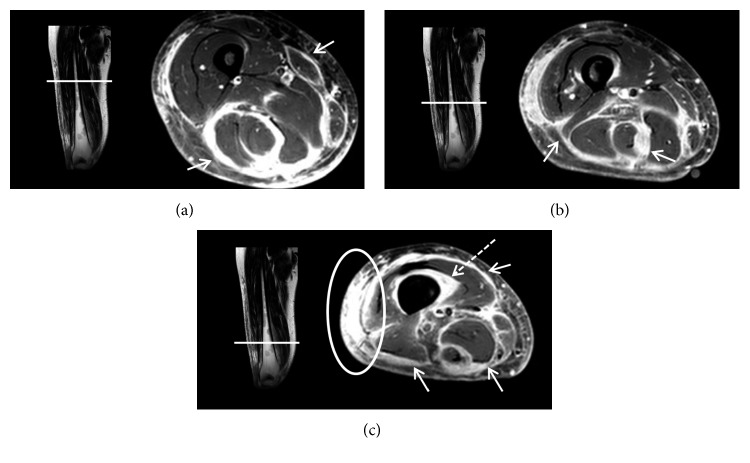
Coronal STIR and axial T1-weighted postcontrast SPIR magnetic resonance images of the upper (a), middle (b), and lower (c) right thigh. The images show considerable and diffuse inflammatory changes along the fasciae of all muscles of the right lower thigh (arrows) and surrounding the cortical bone of the distal femur (dotted arrow), and severe inflammatory changes surrounding the vessels of the popliteal fossa and subcutaneously at the knee level extending cranially along the lateral side of the thigh (circle).
